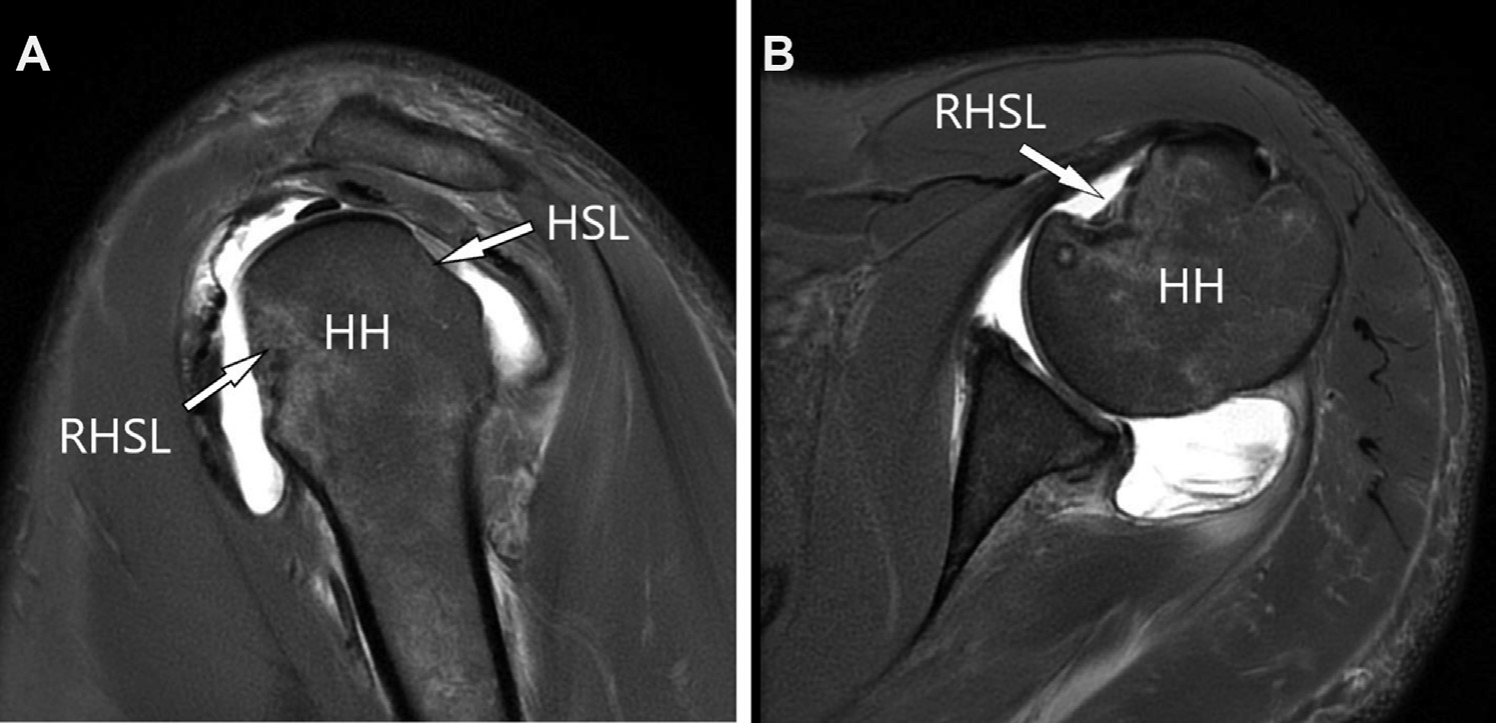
Shoulder MRI showing lesions. (A) Sagittal plane: simultaneous acute HSL and RHSL after traumatic injuries. (B) Axial plane: RHSL. There are no relevant posterior glenoid defects, and the anterior glenoid defect is <20%. Abbreviations: HH, humeral head; HSL, Hill-Sachs lesion; MRI, magnetic resonance imaging; RHSL, reverse Hill-Sachs lesion.
